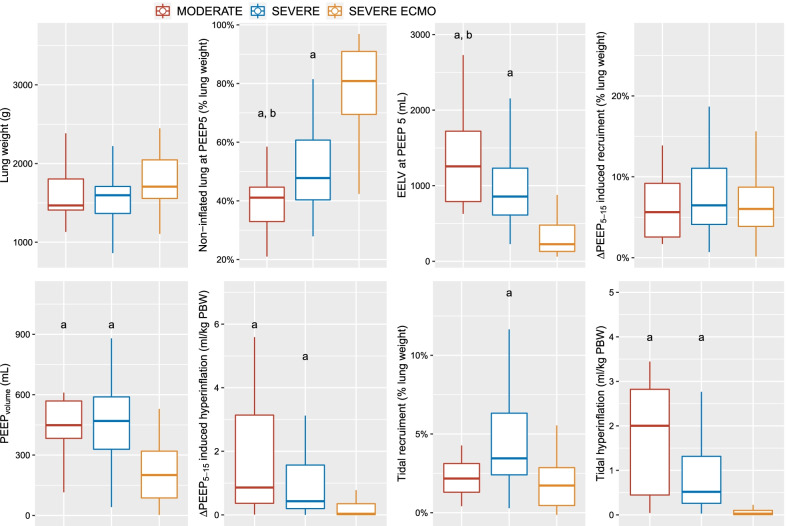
CT parameters as a function of ARDS severity ^a^, *p* < 0.05 vs. severe ARDS with ECMO, ^b^, *p* < 0.05 vs. severe ARDS without ECMO. ARDS, acute respiratory distress syndrome, ∆_PEEP5-15_, change in PEEP from 5 to 15 cmH_2_O, PEEP_volume_, change in lung aeration induced by PEEP change from 5 to 15 cmH_2_O, ECMO, extracorporeal membrane oxygenation, EELV, end-expiratory lung volume, MODERATE, moderate ARDS, PBW, predicted body weight, PEEP, positive end-expiratory pressure, SEVERE, severe ARDS without ECMO, SEVERE ECMO, severe ARDS under ECMO
